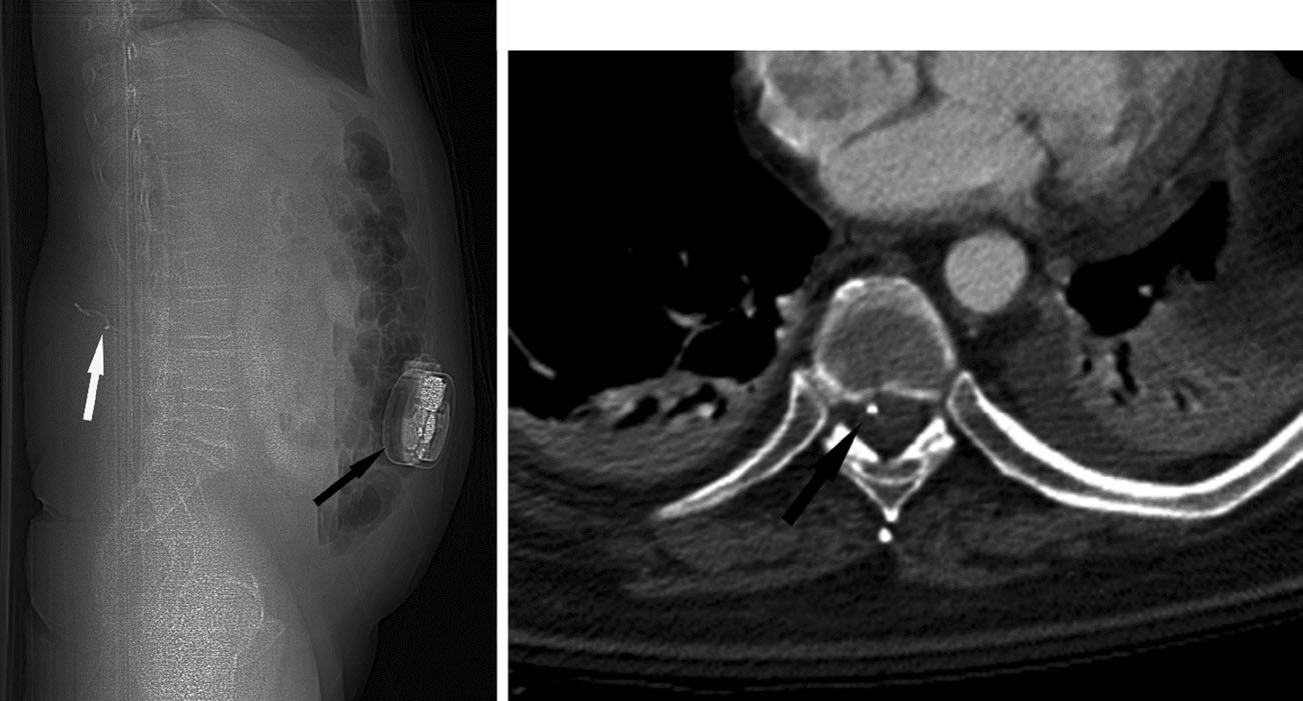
a The lateral scout image from an abdominal CT of a 39-year-old male with C2 SCI from a fall 4 years prior shows an intrathecal baclofen pump in the right lower abdominal quadrant (*black arrow*). The tubing from the pump extends posteriorly (*white arrow*) before entering the thecal canal. b Axial CT image of the same patient confirms the intrathecal position of the catheter tip (*black arrow*)
